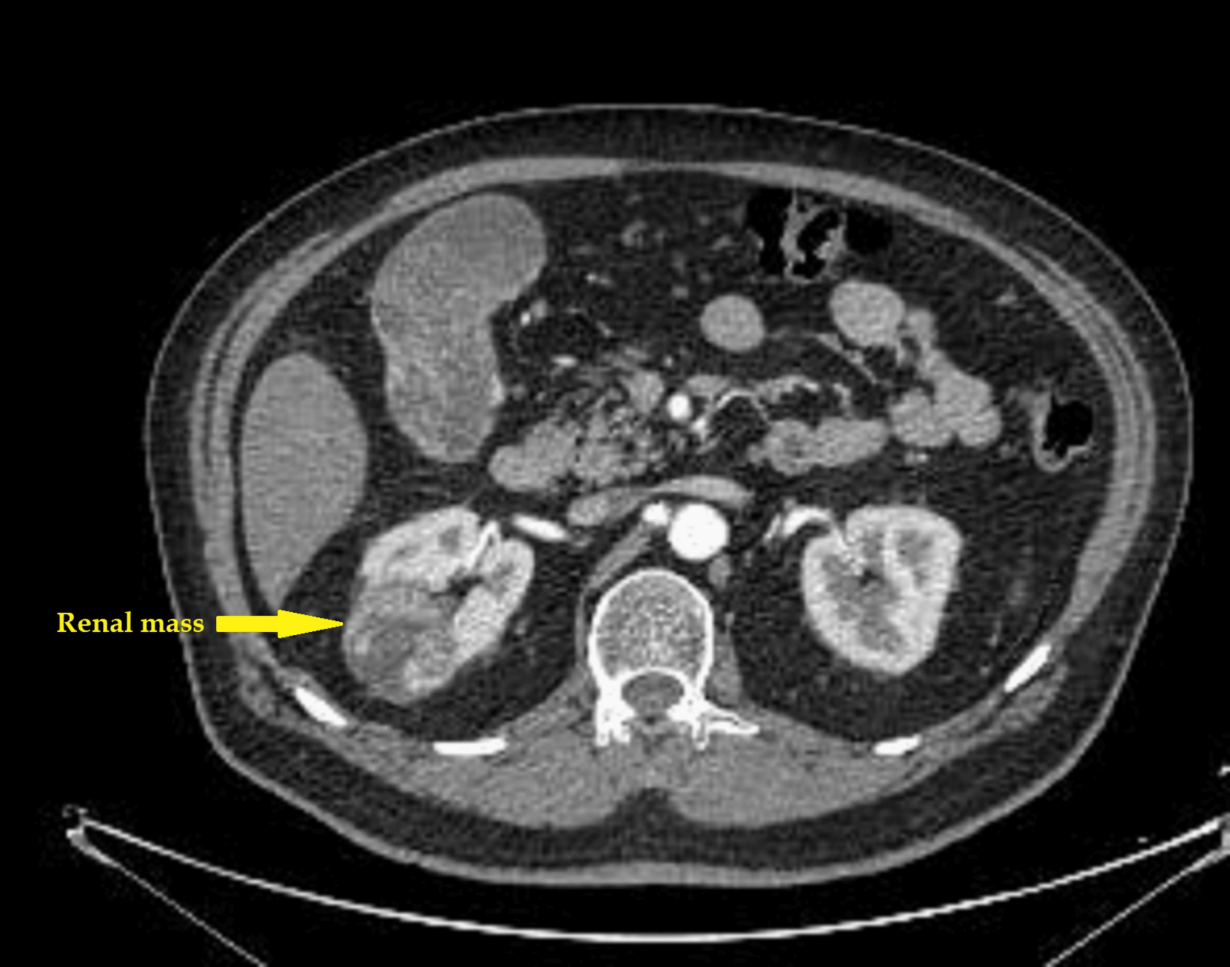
A CT scan showing a right renal upper pole mass (arrow), an inhomogeneous enhancing partially exophytic mass 43 mm in greatest dimension involving the posterior part of the upper pole. The nephrometry score was 8P, highly suggestive of renal-cell carcinoma.
